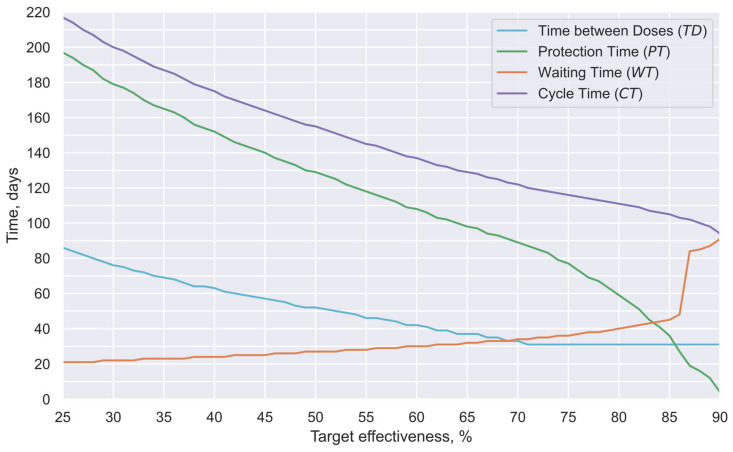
Pareto frontier with the results of the optimization. Recommended time between doses for each effectiveness (TD, cyan curve), the period of time under protection (PT, green curve), the number of days between the first dose and first day under the target protection (WT, orange curve), and the number of days between the first dose and last day under the target protection (CT, purple curve).
